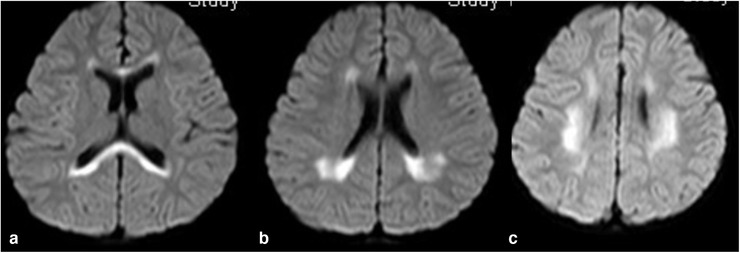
Cranial MRI revealed lesion extending to other areas of the corpus callosum (a), adjacent peri ventricular (b), and bilateral semi‐ovoid center (c)
